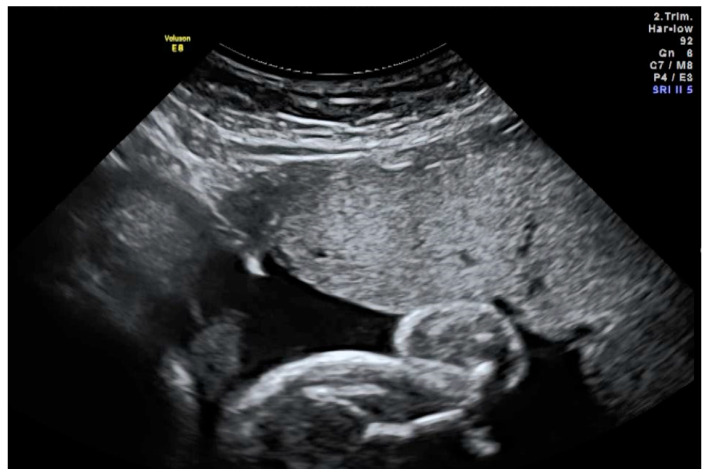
Ultrasound-guided injection of the study drug into the amniotic cavity. Advancement of the needle must be continuously visualised. In this image, the needle tip (white) is located correctly in the amniotic fluid (black) underneath the placenta.
